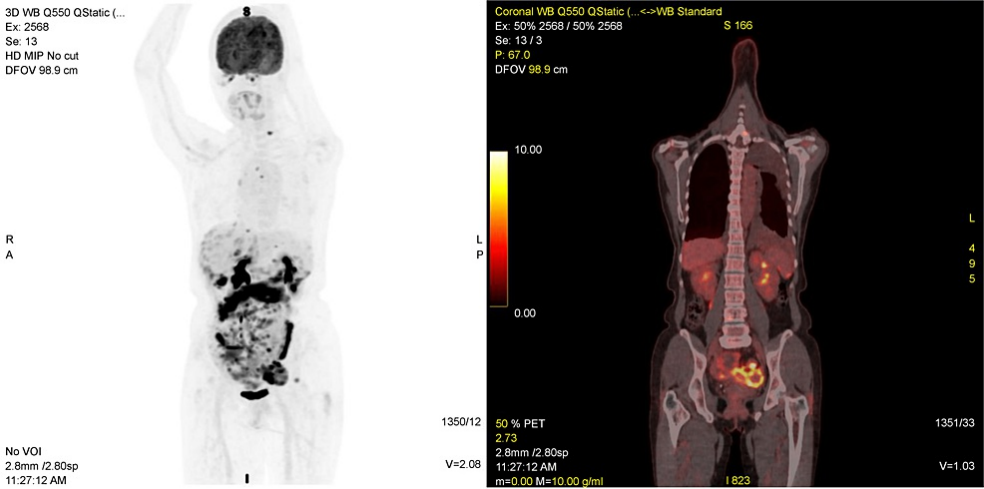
Disseminated FDG-avid foci in the peritoneum, mesentery, celiac LNs, paraaortic LNs, and omentum with a strong FDG-avid omentum cake in the mid-abdomen (SUVmax: 14.3), and cul-de-sac. The lower conspicuity foci were over the left breast. FDG: fluorodeoxyglucose; LN: lymph node
